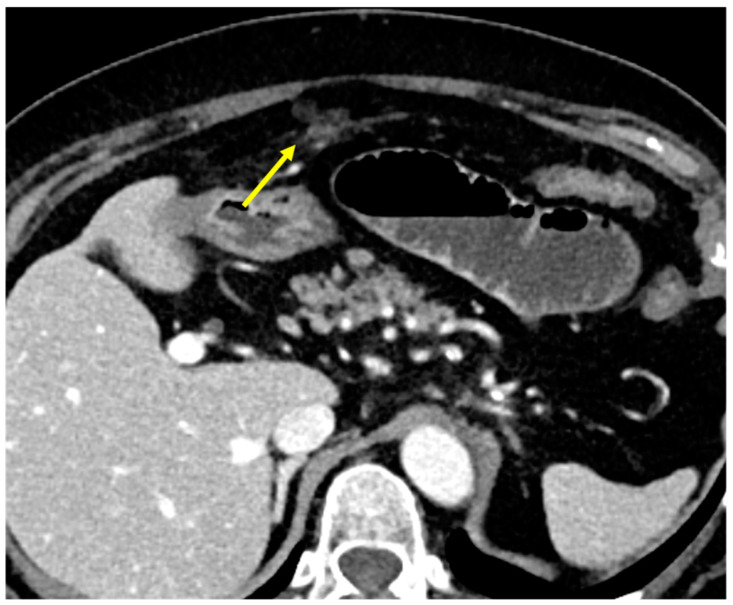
Perigastric appendagitis. A middle-aged woman with mild abdominal pain. At CT, a heterogeneous, fat-density, ovoidal lesion with mild surrounding fat stranding along the course of the gastrohepatic ligament is visible (arrow).
